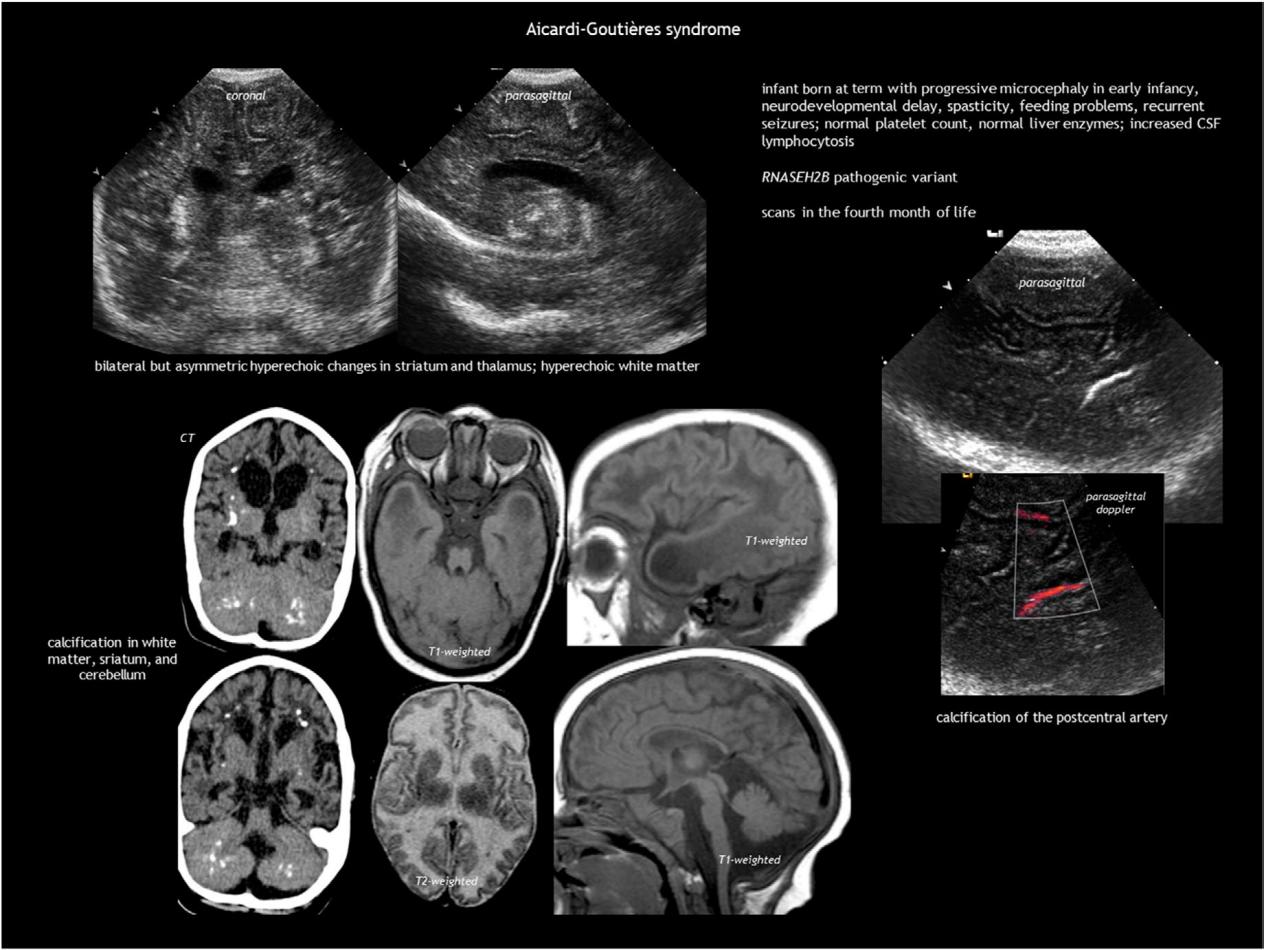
Aicardi–Goutières syndrome. Abbreviations: CSF, cerebrospinal fluid; CT, computed tomography.
